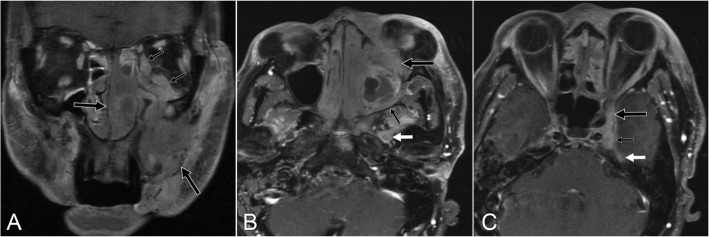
Postcontrast MR images of a 66-year-old-man with NK/T-cell lymphoma who presented with facial numbness (patient 18). a Coronal image shows a mass in the left side of the face and nasal cavity (large arrows) with infiltration of the orbit including the extra-ocular muscles (small arrows). b Axial image shows the orbital mass (large black arrow) and extension through the inferior orbit fissure to the pterygopalatine fossa (small black arrow). Involvement of the mandibular nerve (V3) is also present (white arrow). c Axial images shows involvement of the cavernous sinus (large black arrow) and Meckel’s cave (small black arrow). There is spread via the superficial petrosal nerve (white arrow) to the facial nerve (not shown)
